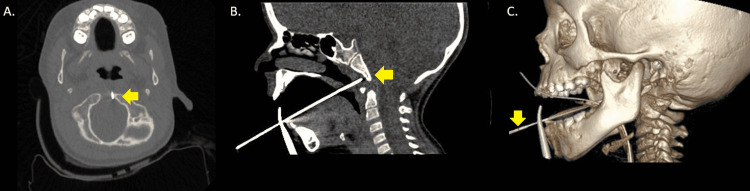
Transoral biopsy of the clivus (A) Axial intraoperative CT at the level of the foramen magnum; (B) Sagittal intraoperative CT at the midline; (C) Intraoperative 3D reconstruction.
